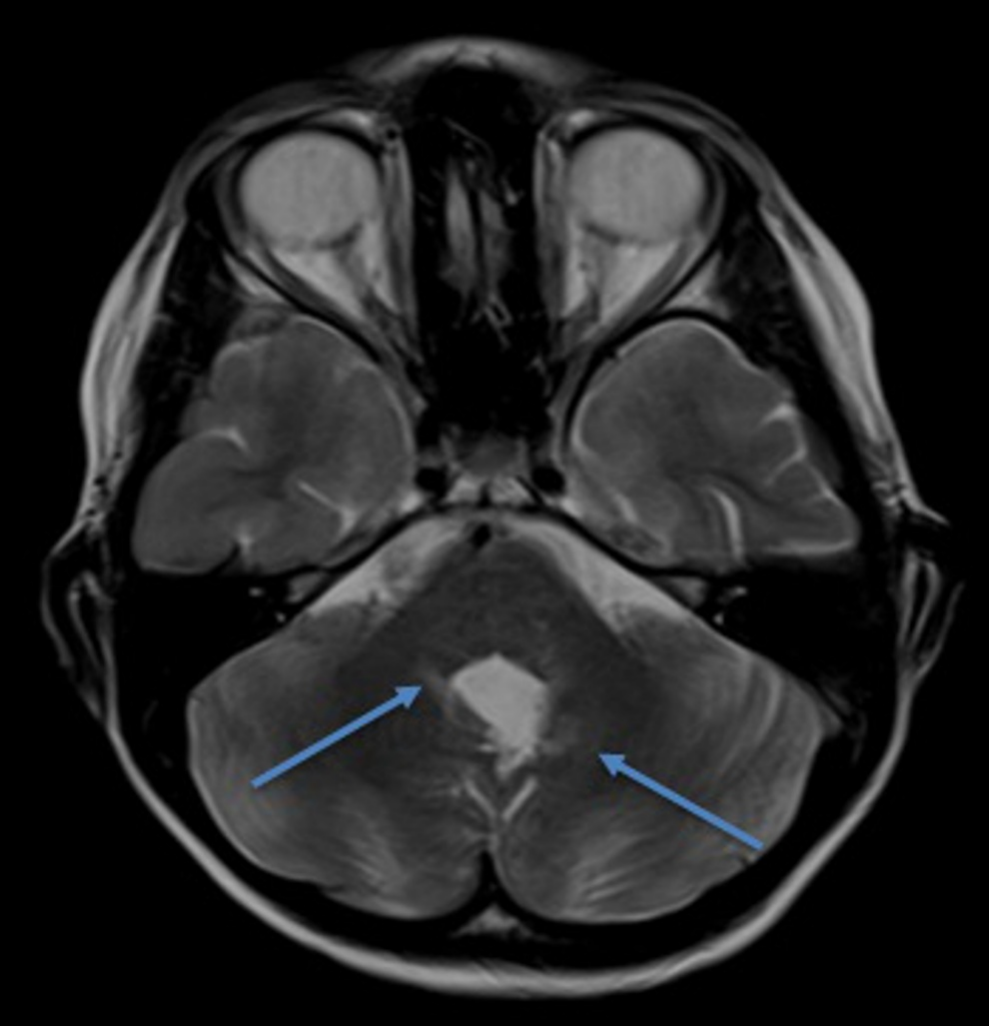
T2-weighted brain magnetic resonance imaging Arrows indicate areas of hyperintensity distant from the surgical field.
